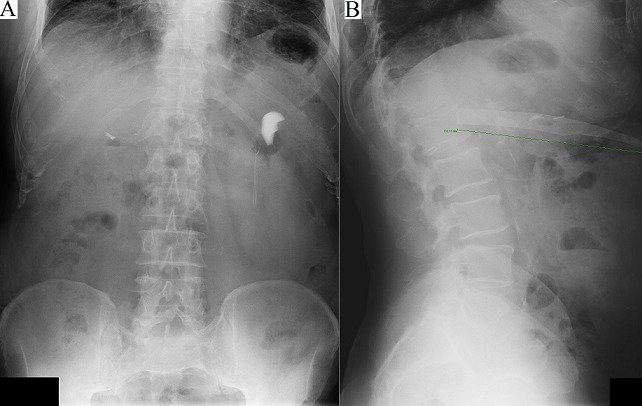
(A) supine abdominal x-ray, January 2014; (B) lateral abdominal x-ray, April 2015
